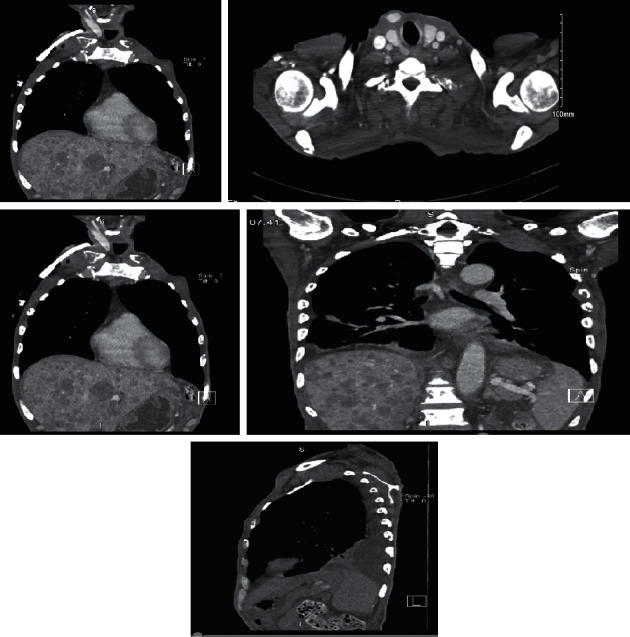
Images of the chest CT scan showing metastasis to the liver and chest wall.
